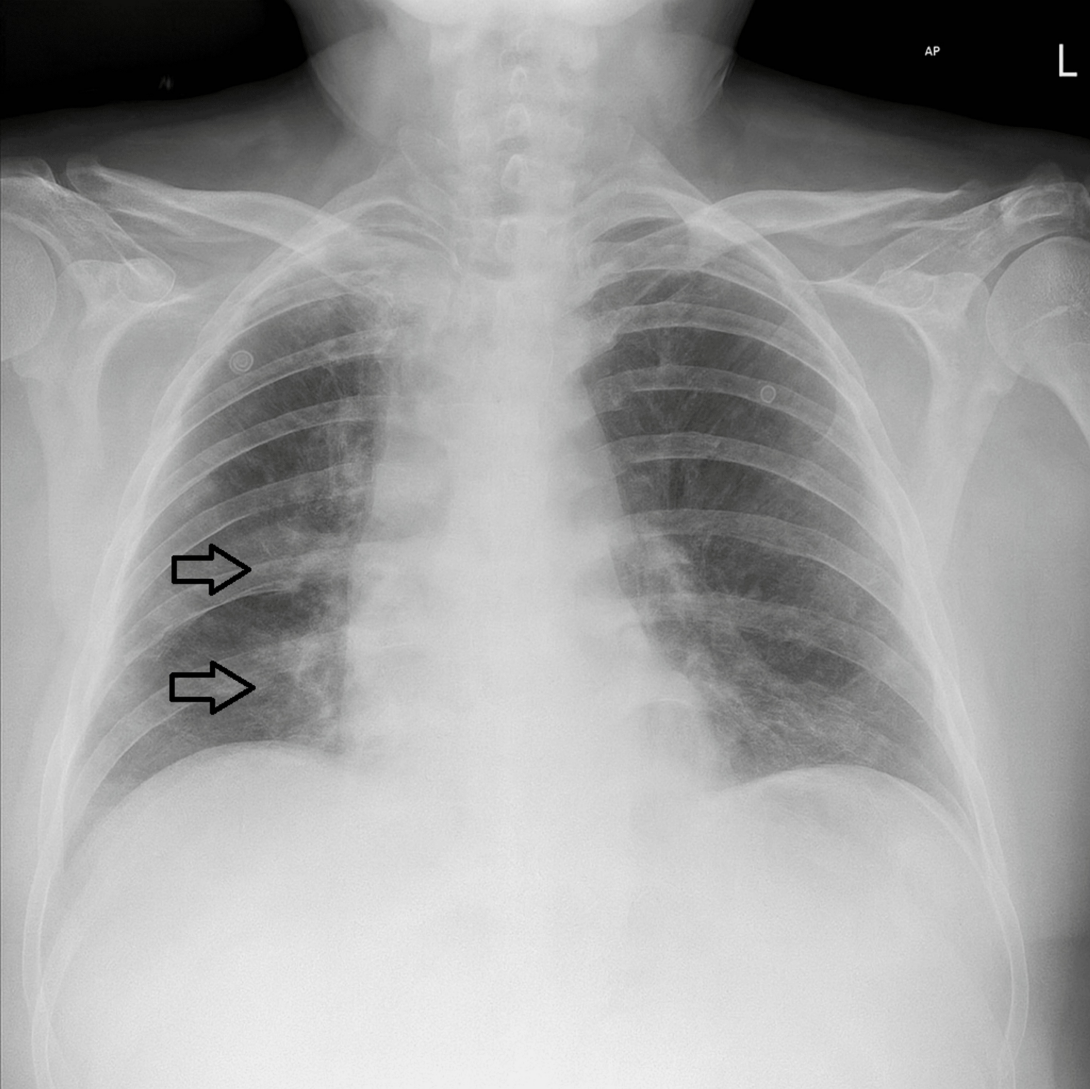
Chest X-ray showing right-sided mid-to-lower zone air-space opacification, consistent with aspiration pneumonia.
